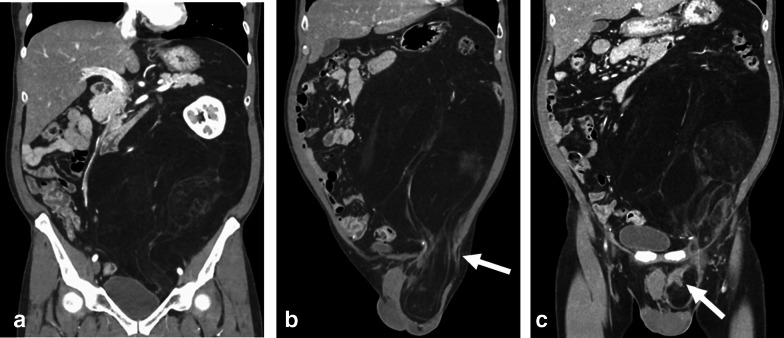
a. Contrast-enhanced CT imaging exhibited a substantial adipose-dense tumor originating from the caudal aspect of the left kidney. b. The tumor has been extending through the left inguinal canal, and reaching the scrotum (indicated by arrows). c. Notably, the mesh plug that had been implanted one year earlier was visibly protruding due to the presence of the tumor (indicated by arrowheads)
